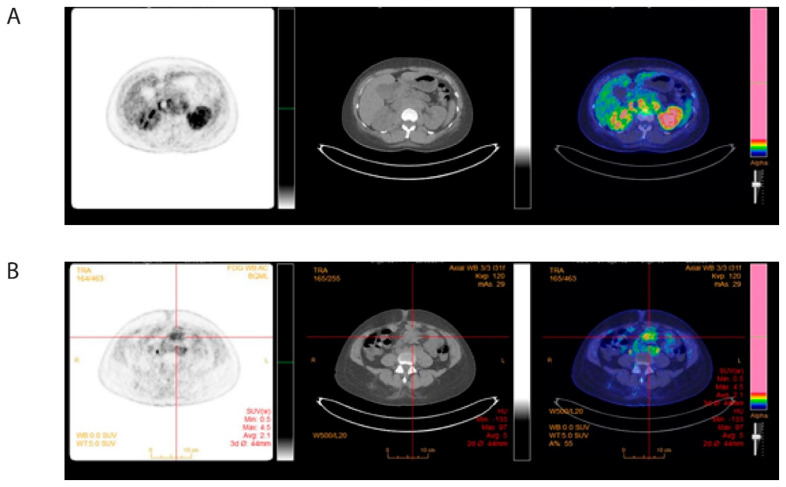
Pretreatment 18-fluorodeoxyglucose (FDG) positron emission tomography (PET)-computed tomography (CT) of abdomen. (A) [^18^F]-FDG-PET/CT at diagnosis showing FDG-positive para-aortic (B) and mesenteric tumor masses.
